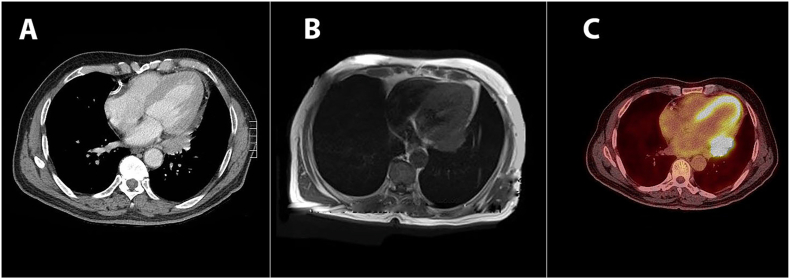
Chest computed tomography (CT) showing posteriorly located pericardial mass (A). Cardiac magnetic resonant imaging (MRI) confirming the location of the mass (B). Positron emission tomography (PET) scan showing high uptake in the pericardial mass (SUV max 7) (C).
